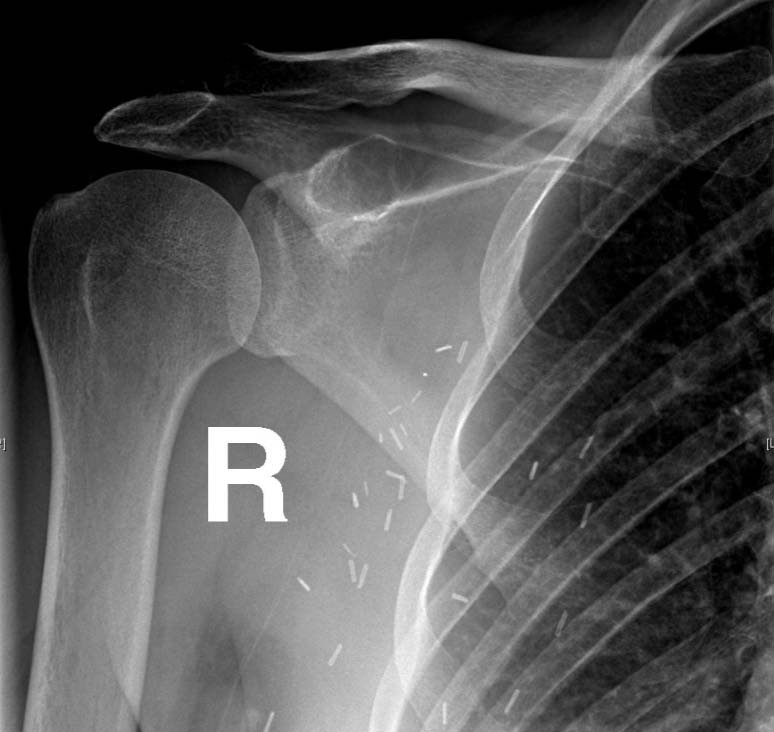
AP radiograph demonstrating the metastatic lesion of the coracoid process.
